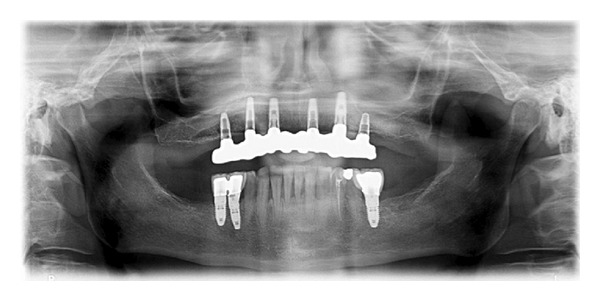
Panoramic radiograph 30 months after loading.
